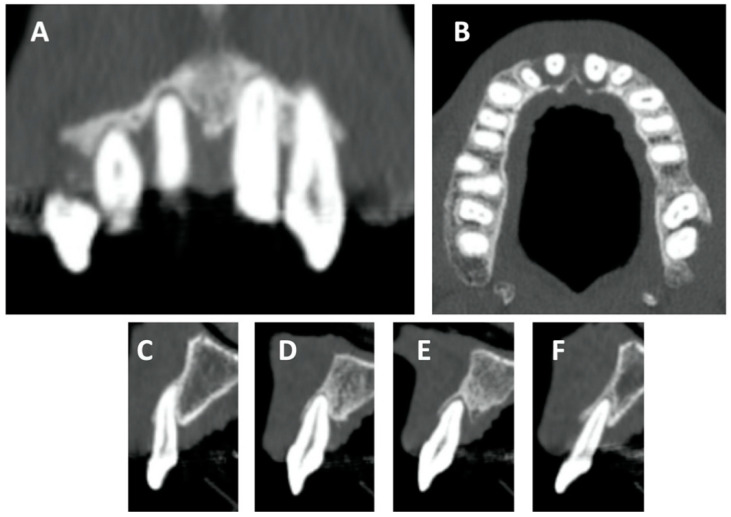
Details obtained from CBCT: (A) superior incisor coronal view, (B) superior incisor axial view, and (C–F) superior incisor sagittal view. Diffused radiolucent areas suggest a low grade of bone attachment, particularly in the area of the superior incisors.
